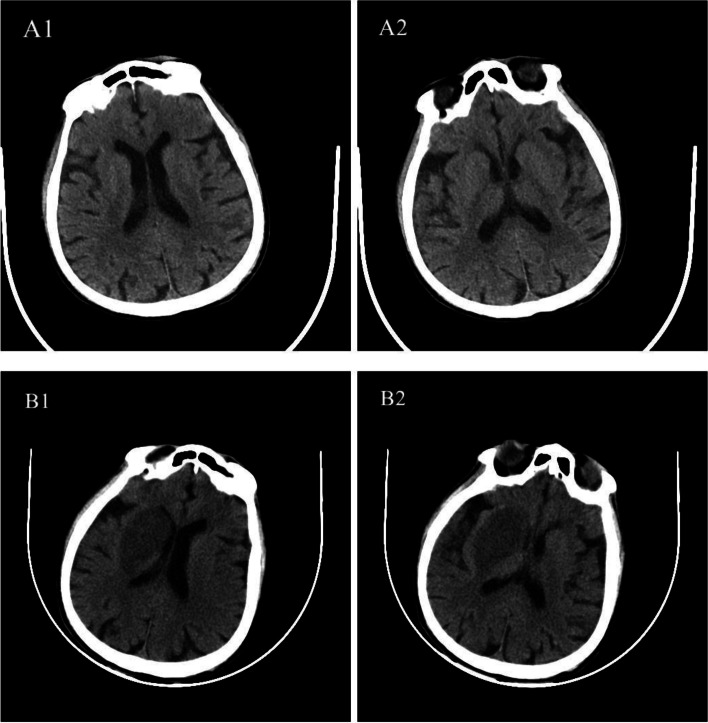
Series head CT scan. A1-A2 scan obtained on illness days 8 showed a normal head CT. B1-B2 scan obtained on illness days 11 showed a low-density shadow in the right basal ganglia
